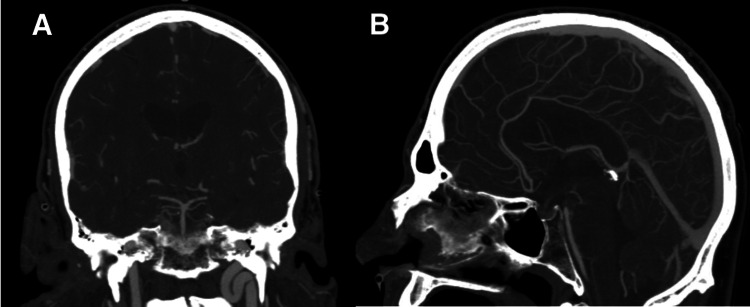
Computed tomography angiography of the head (mean intensity projection) without evidence of basilar perforator aneurysm in the sagittal (A) nor the coronal (B) plane
